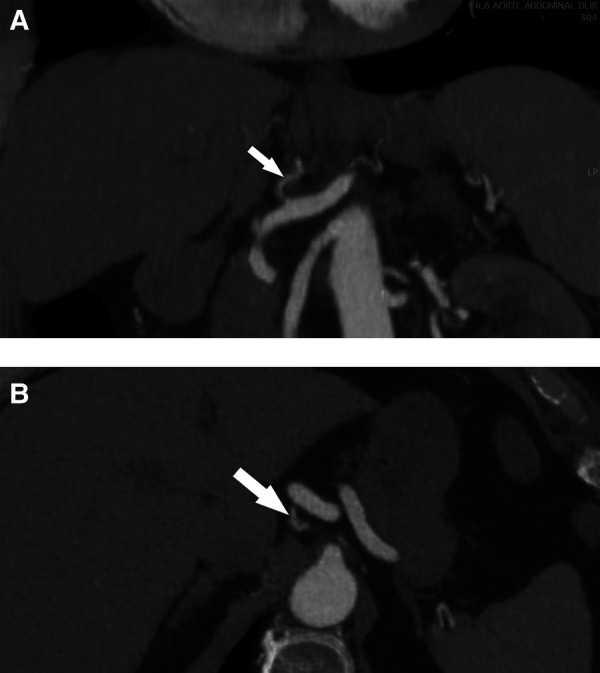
Helicoidal 512 slice computed tomography angiography at 6 months in arterial phase with 1.3 mm slices. (A) Sagittal view of a less-thickened LGA (arrow) with near complete resolution of dissection. (B) Coronal view with a recanalization of the lumen of the LGA (arrow). LGA, left gastric artery.
